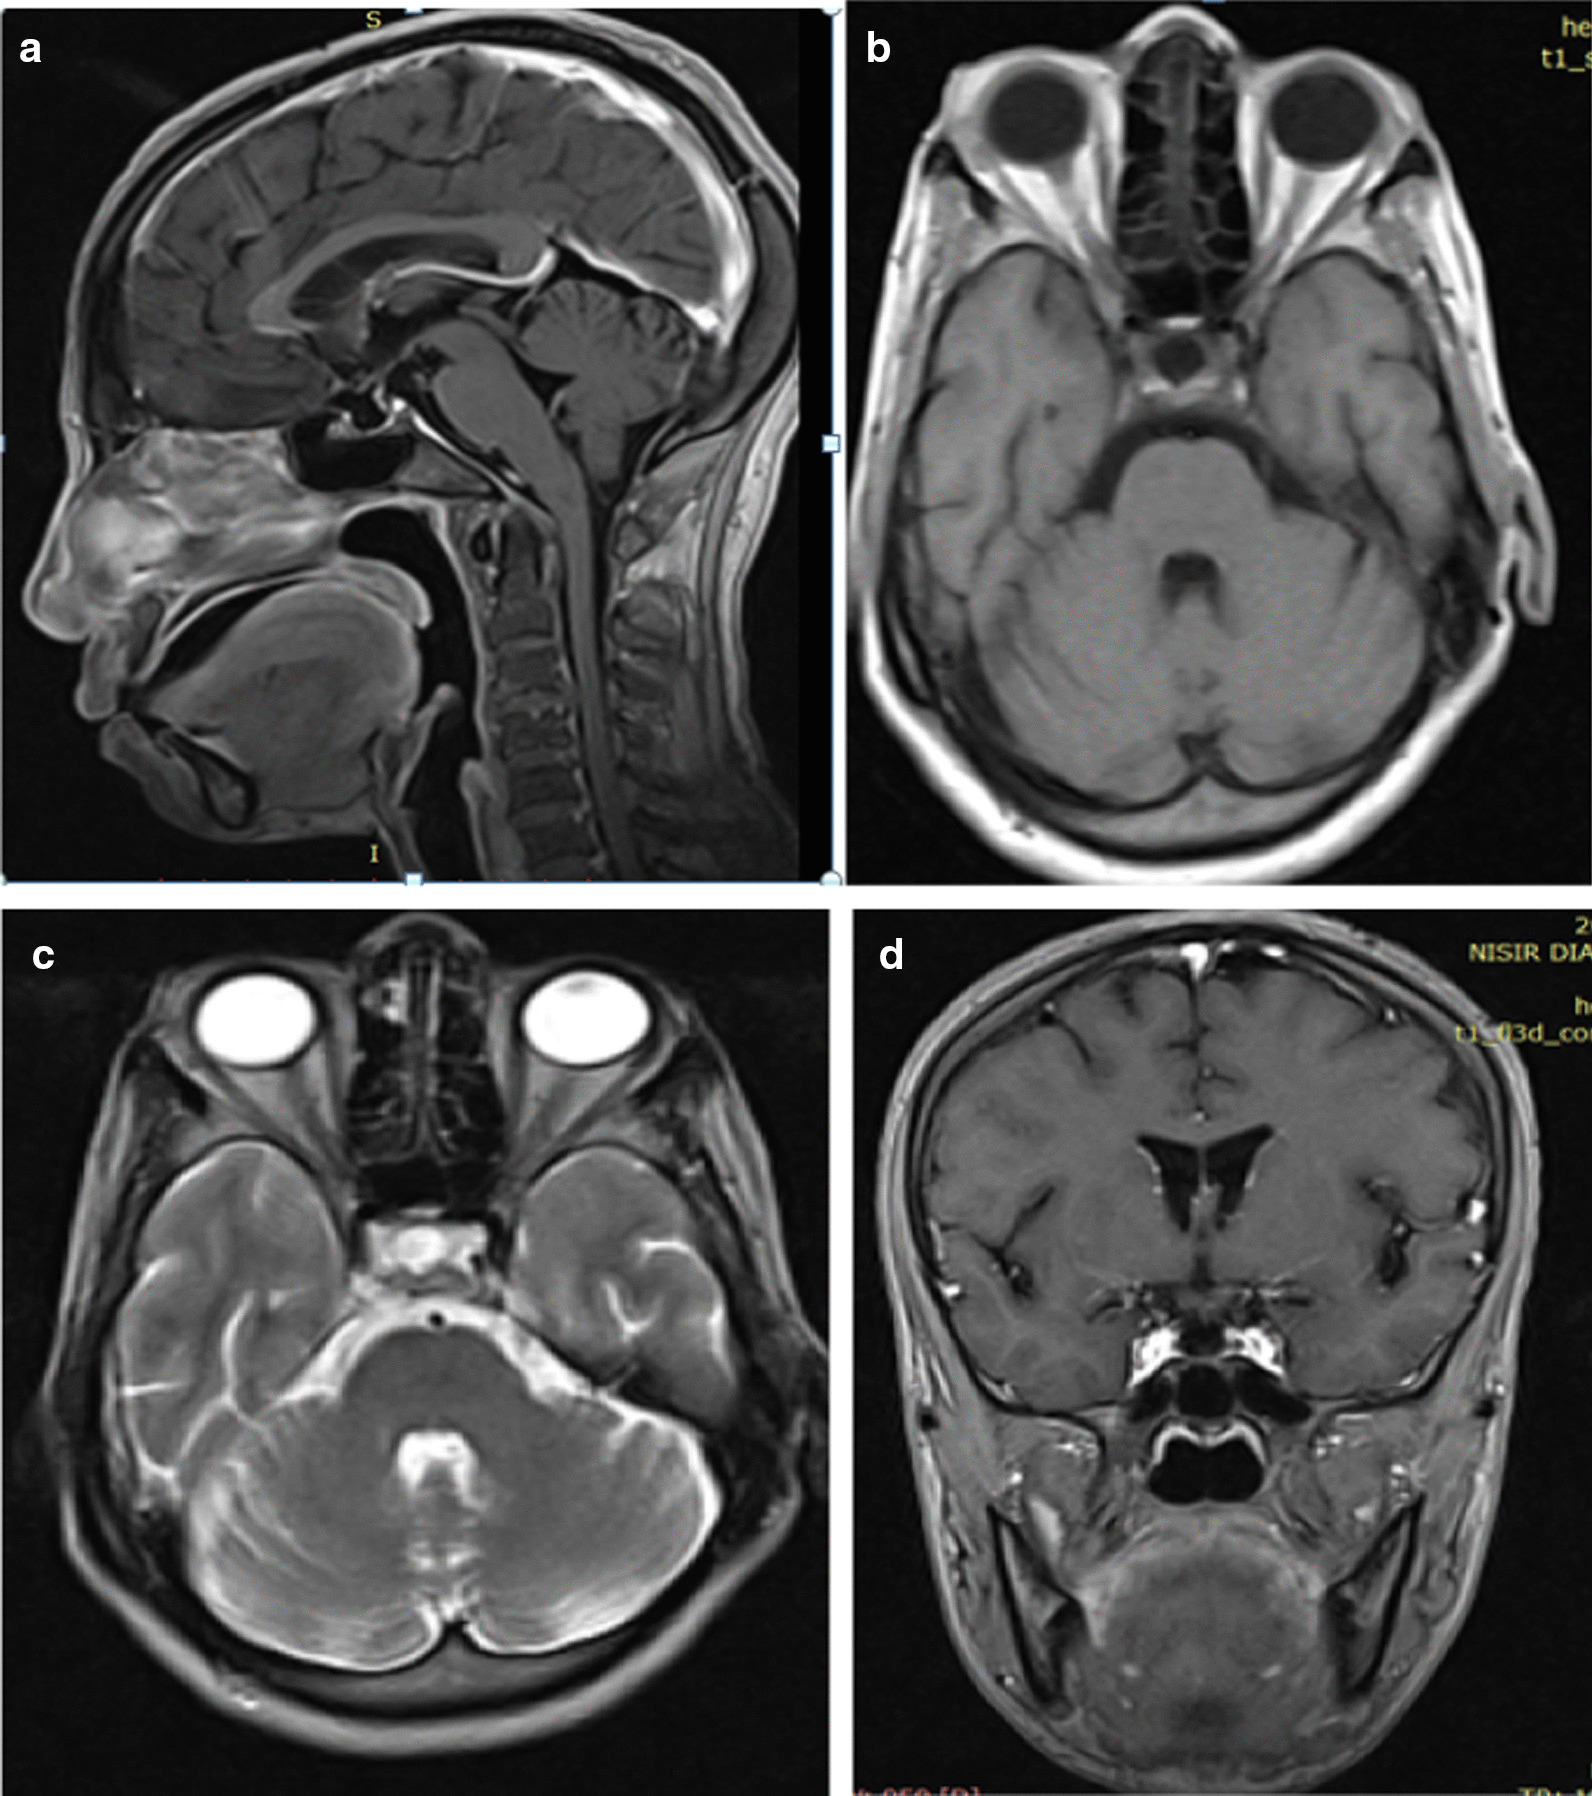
Brain magnetic resonance imaging shows an empty sella. a T1-weighted post contrast, b T1-weighted axial, c T2-weighted axial, d T1-weighted coronal
